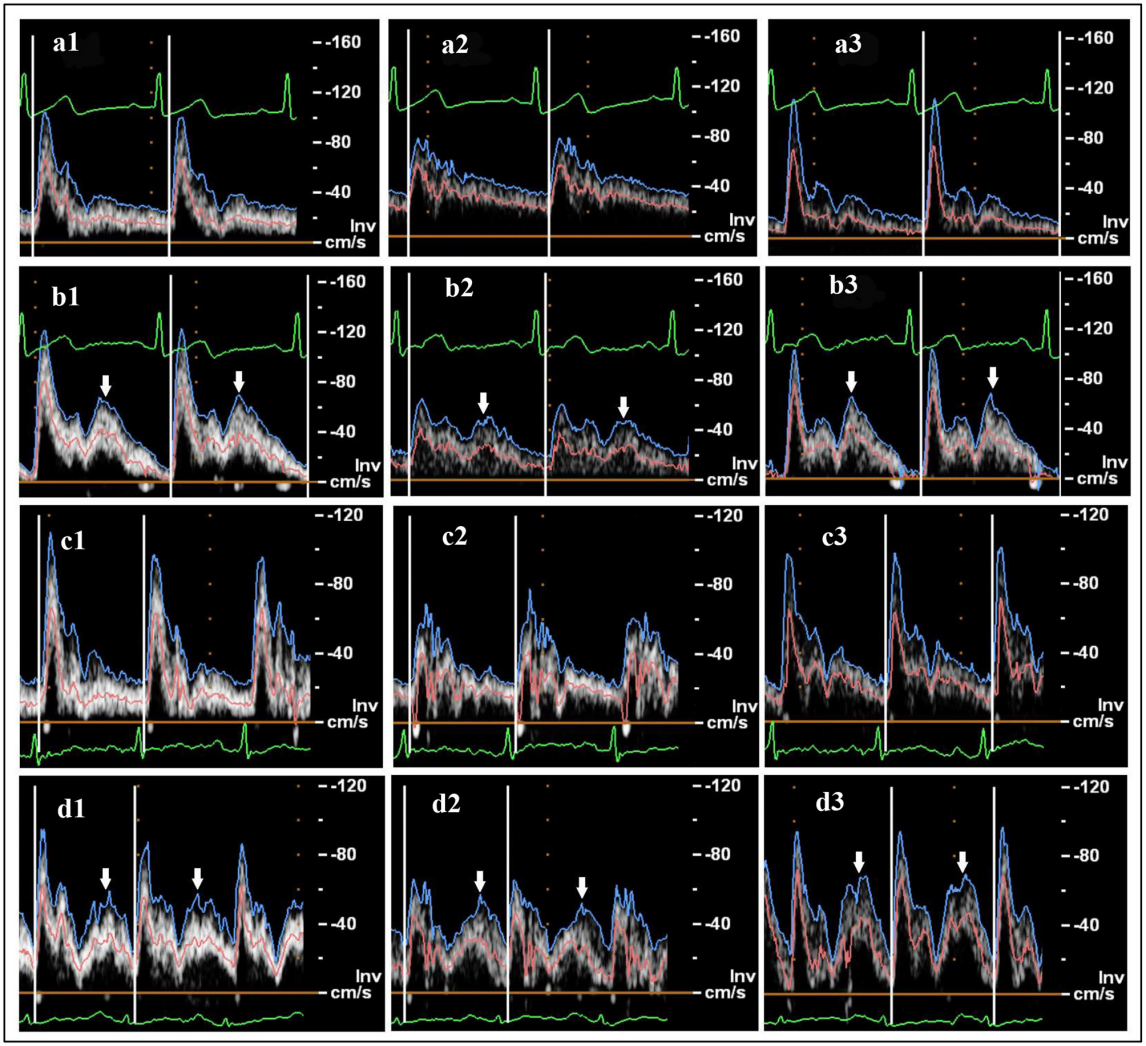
Ultrasound flow velocity spectrum: (a) healthy subject, pre-enhanced external counterpulsation (EECP); (b) healthy subject during EECP with 30 kPa cuff pressure; (c) patient, pre-EECP; (d) patient during EECP with 30 kPa cuff pressure. Common carotid artery (CCA), internal carotid artery (ICA), and external carotid artery (ECA) are represented by 1, 2, and 3, respectively. The blue, pink, and green curves represent the peak flow velocity waveforms, mean flow velocity waveforms, and ECGs, respectively. White arrows indicate the diastolic increase induced by EECP stimulation.
